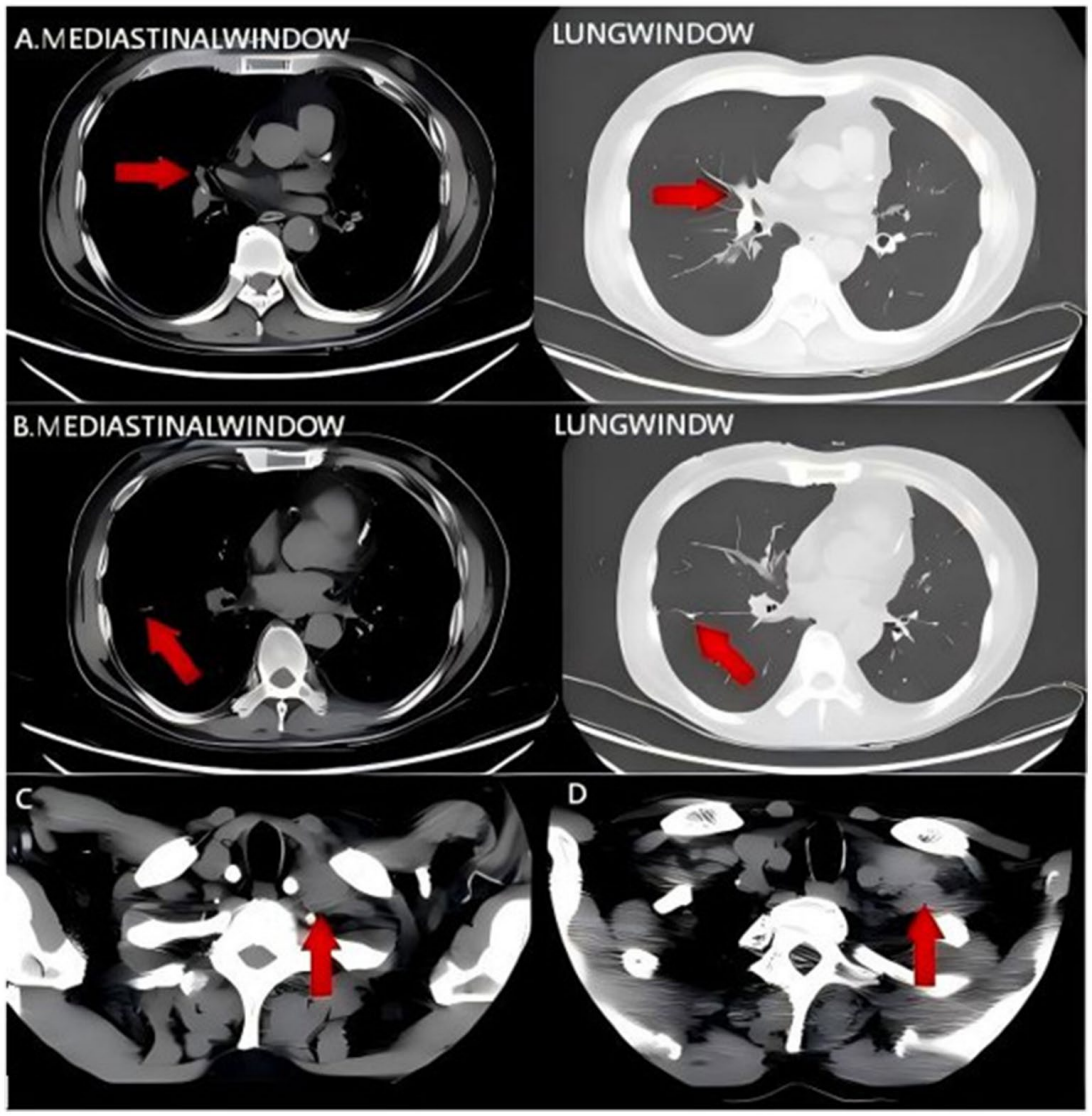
(A–C) Contrast-enhanced CT findings of the neck and chest on June 25, 2025. Enlarged left supraclavicular and mediastinal lymph nodes. (D) CT findings of the neck on August 19, 2025. Stable disease of the lymph nodes. Red arrows indicate the location of the tumor.
